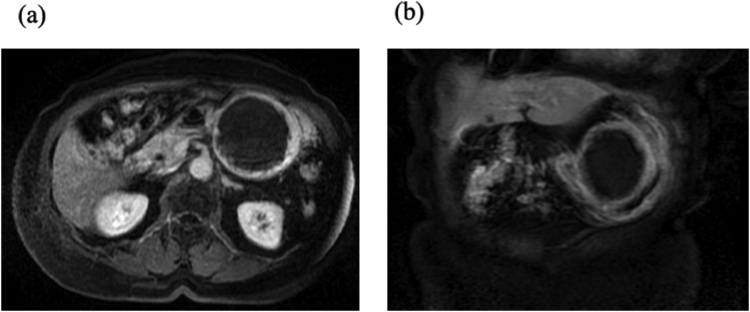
Gadolinium-enhanced abdominal MRI. a/b. There is a smooth-surfaced tumor of 70 × 70 mm at the lesser curvature of the middle stomach and almost all parts of the tumor had a high intensity on T2WI. The submucosal tumor had relocated to the original position.
